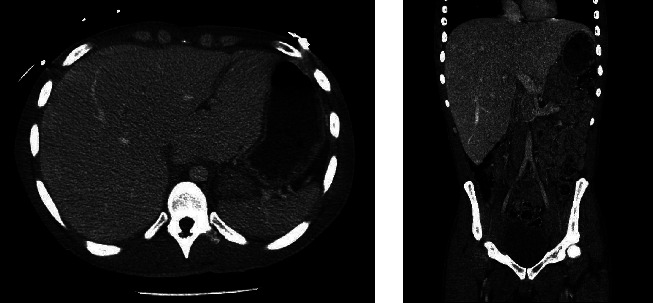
Axial and coronal sections of the abdominal CT scan showing hepatomegaly with a hyperdense liver.
